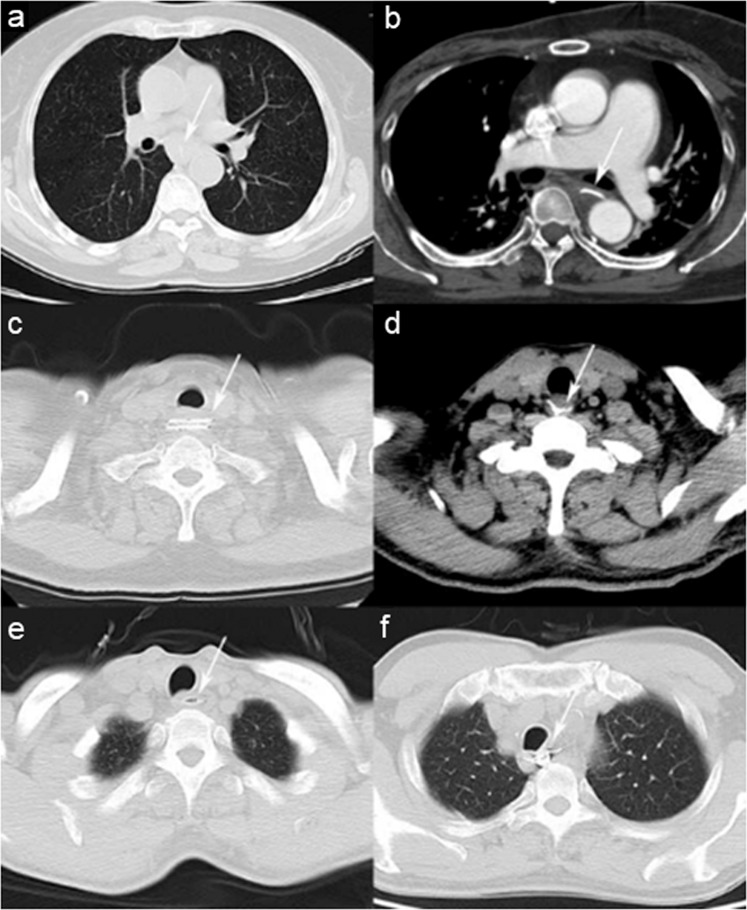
Computed tomographic findings of different types and shapes of esophageal foreign bodies. (a) Pin-shaped fish bone lodged in the mid-esophagus in an anterior–posterior orientation. (b) Pin-shaped fish bone lodged near the aorta in a left–posterior orientation. (c) Sheet-shaped pork bone lodged in the upper esophagus in a left–right orientation. (d) Trident-shaped duck bone lodged near the entrance of the esophagus. (e) Spindle-shaped jujube pit lodged in the upper esophagus in a left–right orientation. (f) Irregular-shaped denture lodged in the upper esophagus.
